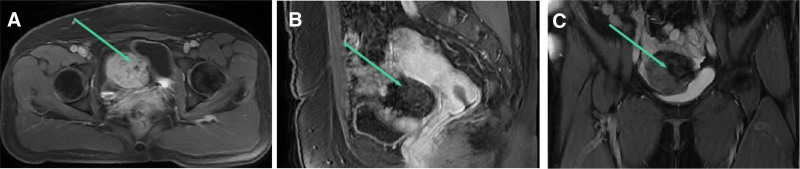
MRI findings. (A) The cross-sectional enhanced scan showed that most of the lesions showed significant enhancement, with no patchy or unenhanced areas visible, and the lesion boundaries were clear; (B) The sagittal position of T1 enhanced scan shows a patchy unenhanced area in the upper part of the lesion, while a clearly enhanced area can be seen in the lower part of the lesion, and the boundary between the posterior part of the lesion and the uterus is clear; (C) T1 enhanced coronal scan shows a patchy unenhanced area in the upper left part of the lesion, with obvious enhancement visible in the right part of the lesion. The boundary between the upper right and the right appendix is unclear.
